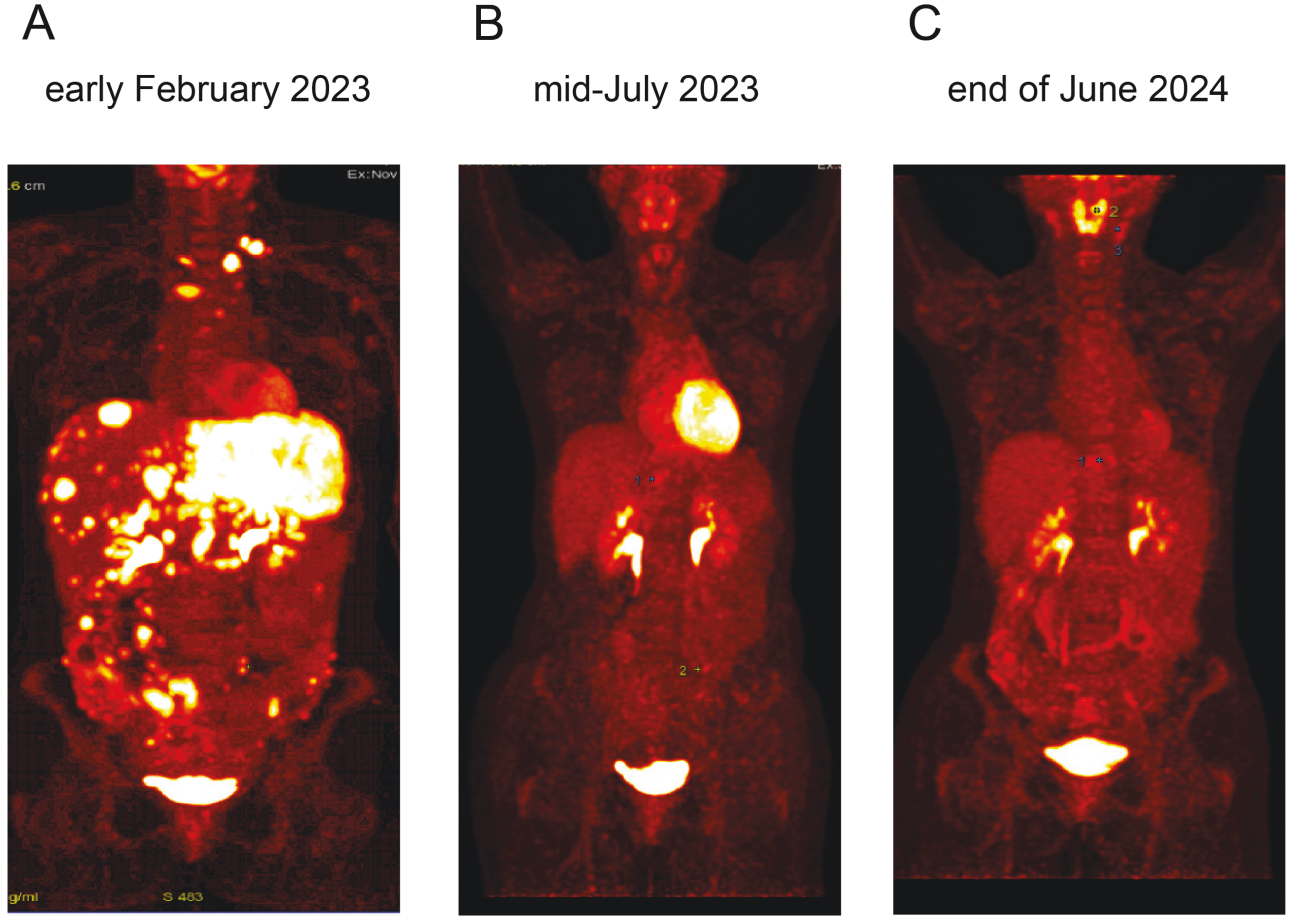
FDG–PET–CT after the failure of first-line therapy with Durvalumab/Cisplatin/Gemcitabine (A) and with a near complete response after 5 months employing Tepotinib (B) and a sustained complete response observed at a follow-up more than 16 months on treatment (C).
